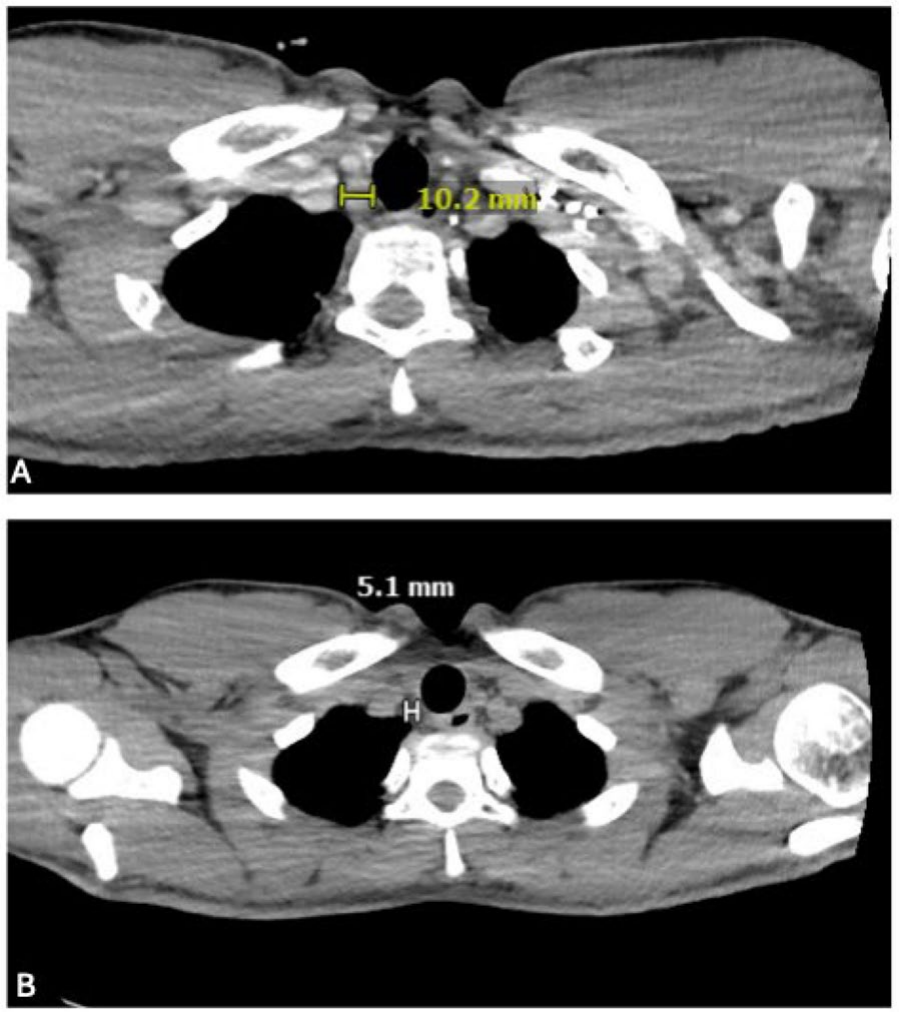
A Computed tomography with contrast chest at time of diagnosis showing 10 mm right paratracheal mass. B A total of 1 year post-treatment, surveillance computed tomography chest revealed a 5 mm right paratracheal soft tissue nodule at site of previous mass
